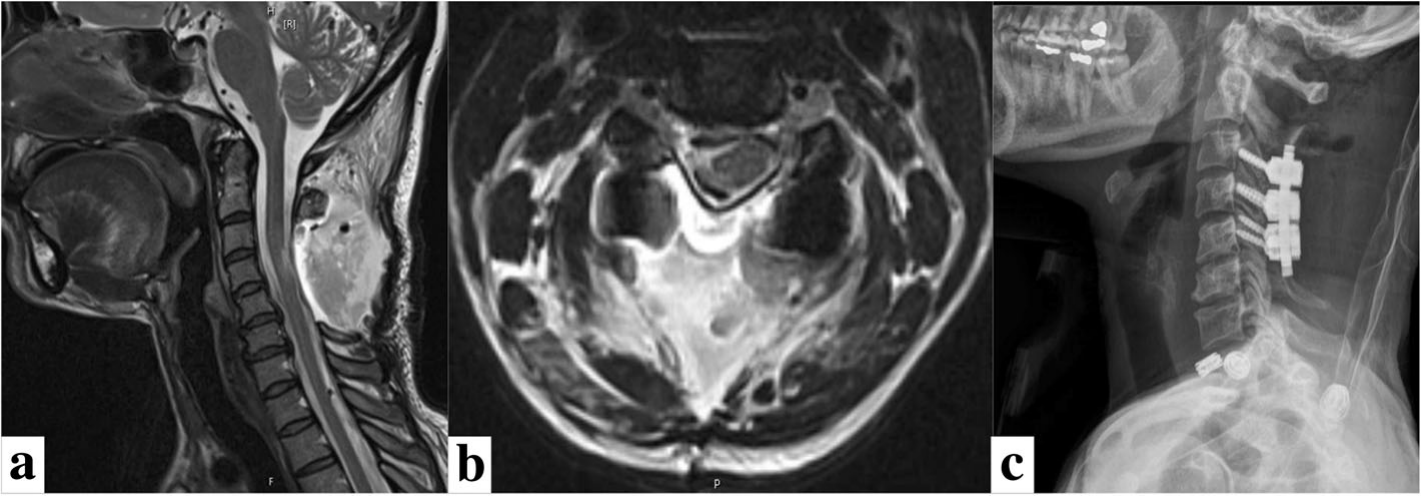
a Sagittal and b axial postoperative T2 MRI. c Lateral X-ray showing gross total resection and stable instrumentation
